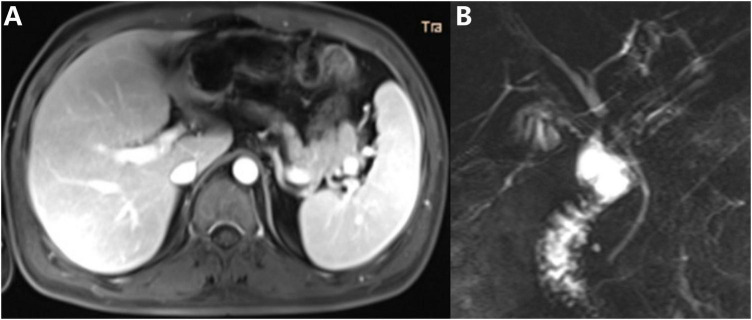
Contrast-enhanced MRI (A) and MRCP (B).
